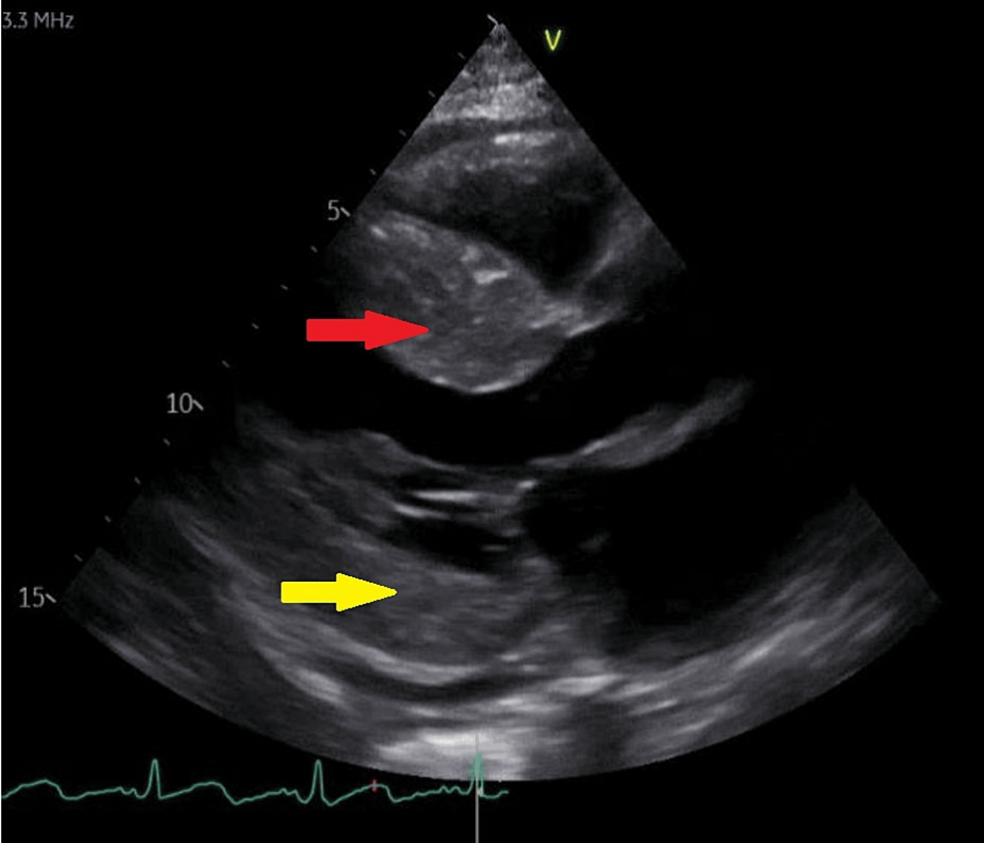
Echocardiogram showing HCM Red arrow: thickened interventricular septum, Yellow arrow: thickened posterior wall HCM: hypertrophic cardiomyopathy
